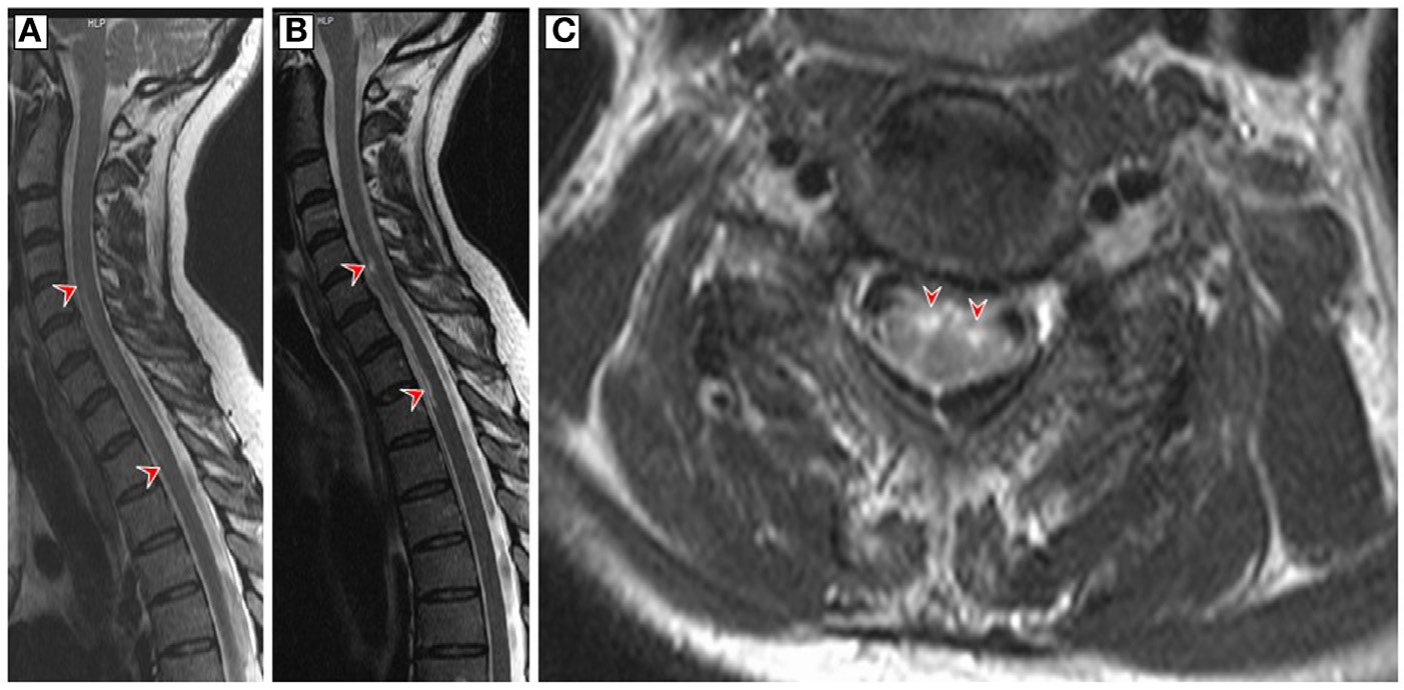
(A) Sagittal T2-weighted magnetic resonance imaging (MRI) of the cervicothoracic spinal cord on admission showed a hyperintense “pencil-like” lesion at the level of the anterior horn which extends from the level of the second cervical to second thoracic vertebral level (red arrowheads) and cord swelling in large parts of the affected area. (B,C) Follow-up T2-weighted MRI 9 weeks later revealed a less homogenous hyperintense filiform signal in the anterior region of the cervicothoracic cord and circumscribed medullar atrophy in the sagittal plane (red arrowheads). The axial MRI is at the C5 level and demonstrates bilateral hyperintensive signals involving the anterior horns of both sides (owl’s eye appearance, red arrowheads).
